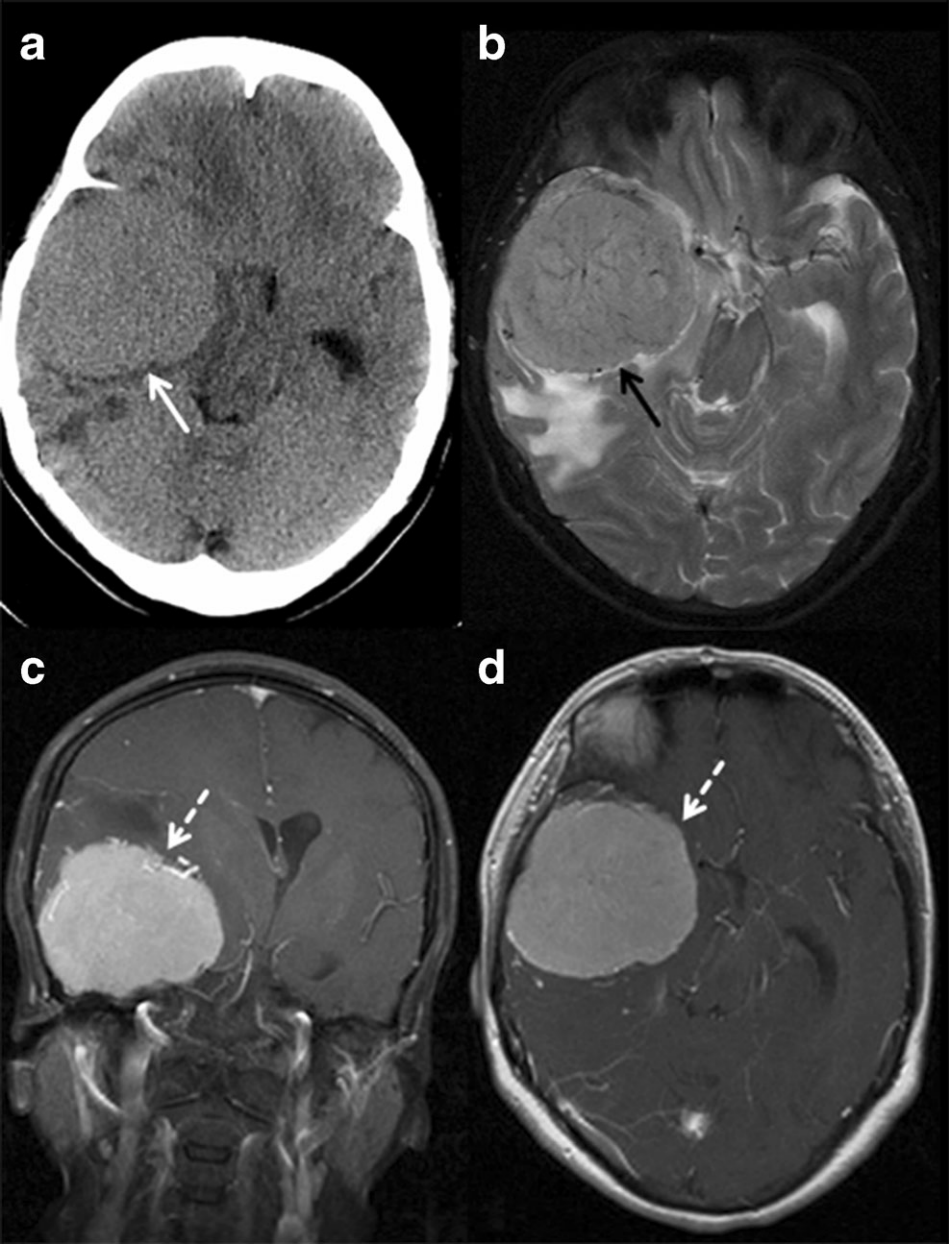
Right middle cranial fossa meningioma. A 55-year-old female presented with LOC and memory disturbance. a axial noncontrast CT section of the brain shows large isodense mass lesion (*white arrow*) in the right middle cranial fossa with significant mass effect. b axial T2W image of the brain at the level of middle cranial fossa shows large extraaxial mass lesion (*black arrow*) in the right middle cranial fossa with perilesional oedema and compression of adjacent right temporal lobe. c & d the lesion is showing homogeneous enhancement (*white dashed arrow*) on post contrast study
